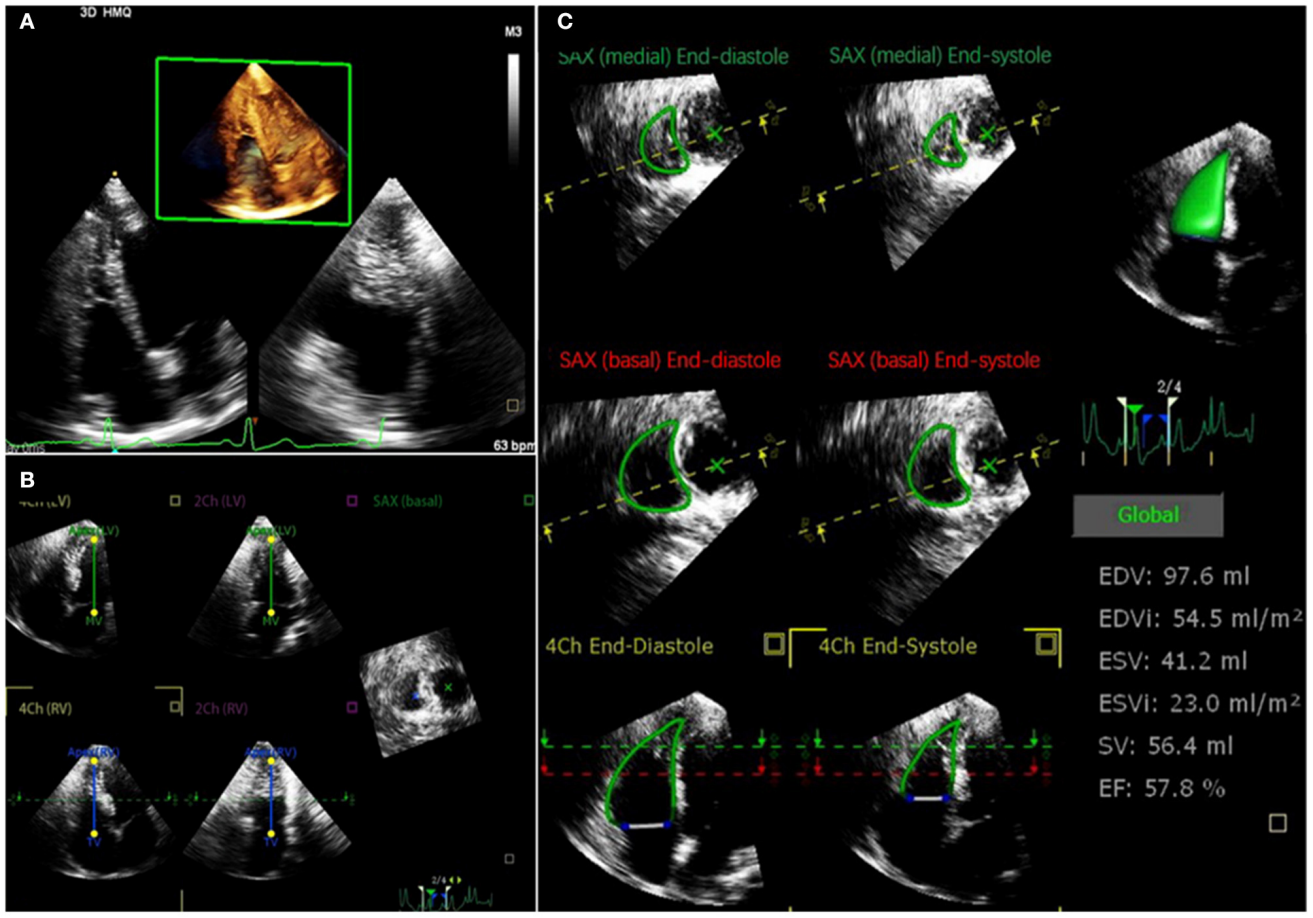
Representative 3DE analysis in a COVID-19 patient. (A) Retrieving 3DE datasets aimed for the RV full-volume images from apical 4-chamber RV-focused view; (B) LV and RV long-axis landmarks in end-diastole in the apical 2- and 4-chamber views were identified by software initially; then, RV focused 4-chamber view and a short-axis view are derived; (C) RV endocardial surfaces were full automatically defined and tracked throughout the cardiac cycle; finally, RV end-diastolic volume, RV end-systolic volume, and RVEF were determined. 3DE, three-dimensional echocardiography; COVID-19, coronavirus disease 2019; LV, left ventricular; RV, right ventricular; RVEF, right ventricular ejection fraction.
